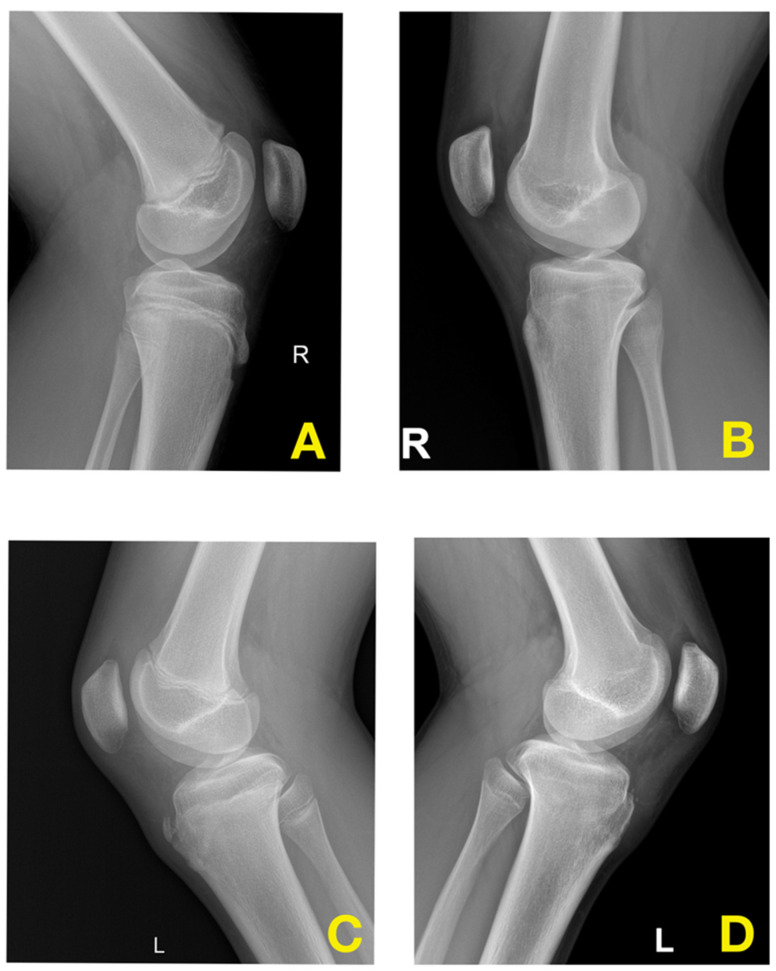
The morphology of the tibial tuberosity area: X-ray images of a patient with acute OSD before (A) and after LR-PRP injection (B) and of a patient with chronic OSD before (C) and after injection (D) after an observation period of 3 years.
